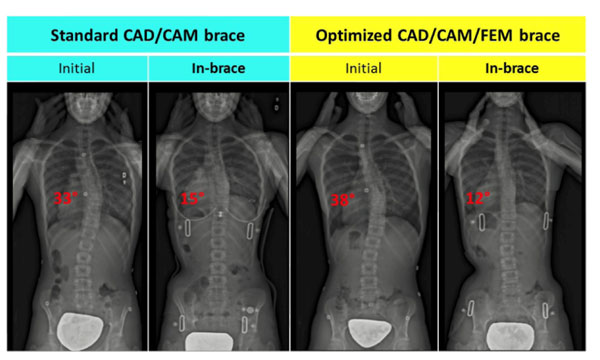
Radiographic results for two typical patients: out of brace for both patients (initial curve), with the StdBrace or with the NewBrace (depending of the randomization), in the postero-anterior view.
